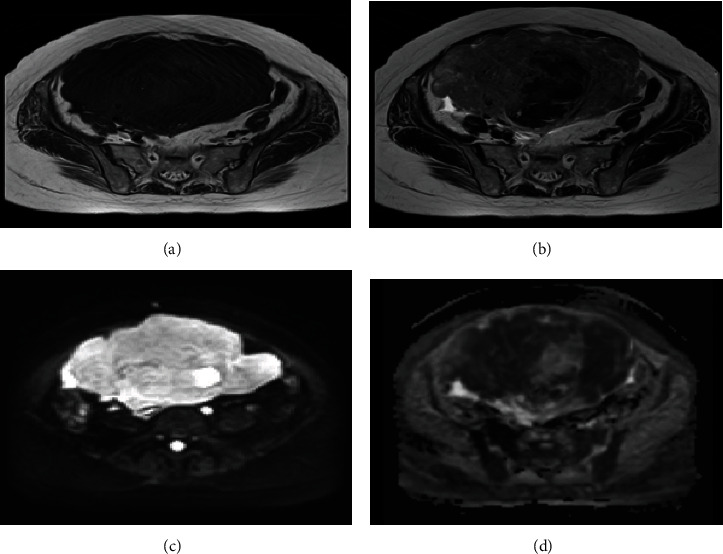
Pelvic MRI imaging: (a) T1-enhanced images; (b) T2-enhanced images; (c) ADC map b = 1000; (d) diffusion-weighted images. The tumor demonstrated moderate to strong T2-enhanced images; marked diffusion-weighted enhancement was noted on the tumor that had almost completely invaded the uterine myometrium.
